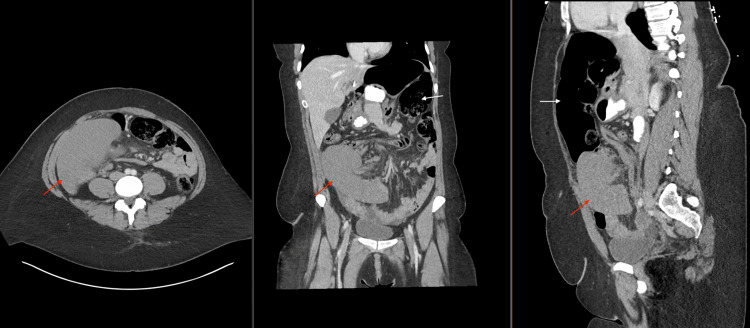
Preoperative CT scan images No free air is seen, and the ischemic spleen is in the right lower quadrant (red arrows). The dilatated and volvulized left colon is shown by white arrows.
